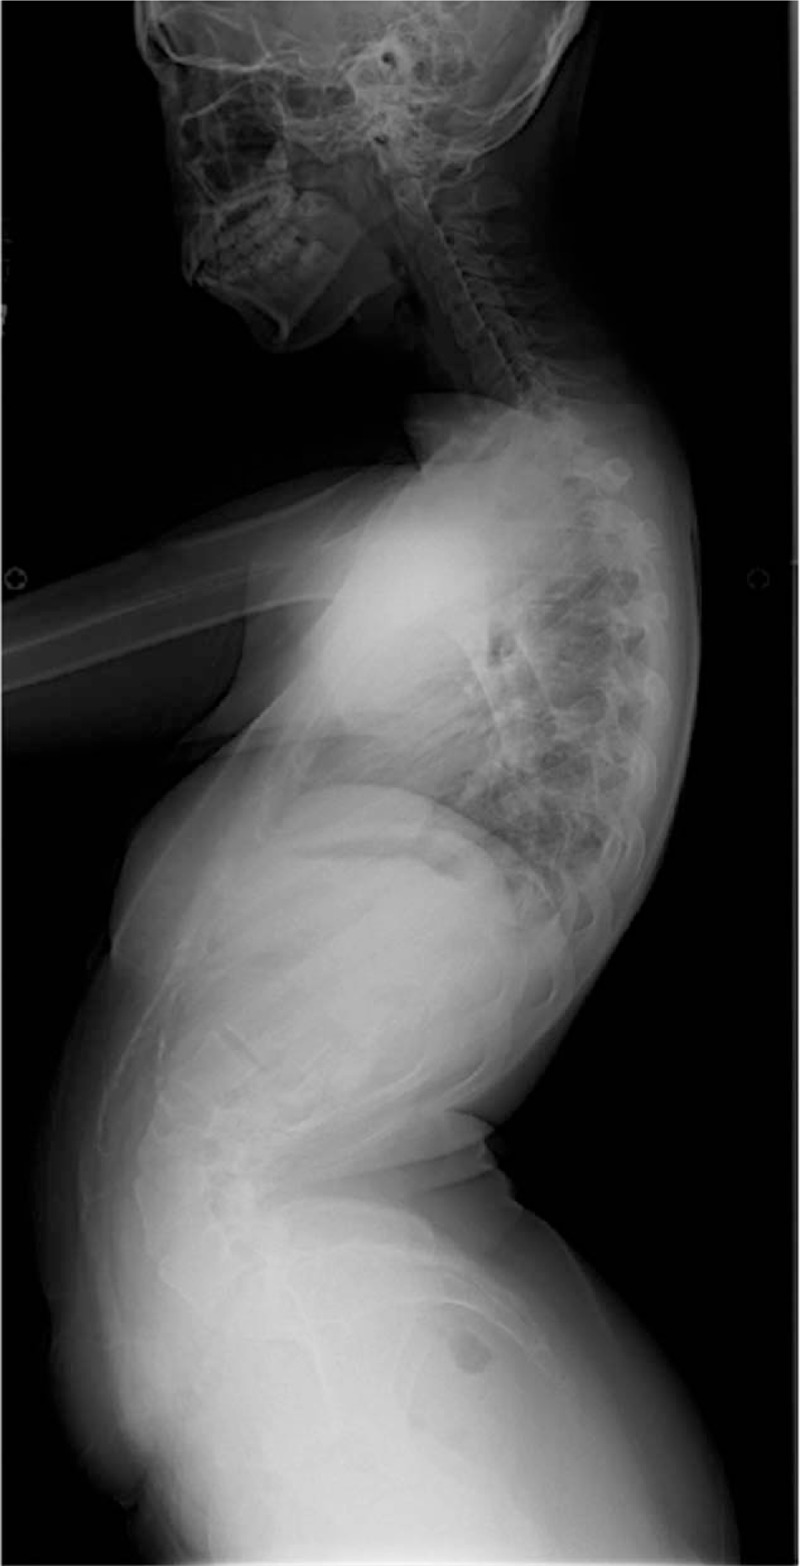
Preoperative lateral radiograph showing a hyperlordosis of 114°, from L1 to L5, and a sagittal imbalance of −100 mm.
